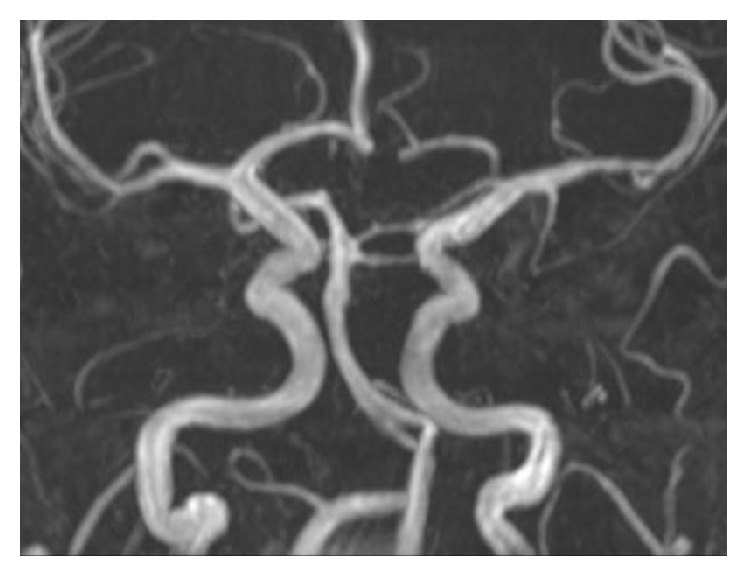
MR angiogram demonstrating patent left MCA. However, if we compare it to the right MCA, there still seems to be some residual stenosis. This MRA was done, a few hours after the initial CT angiogram and at the time patient started to show some fluctuating improvement in his exam.
